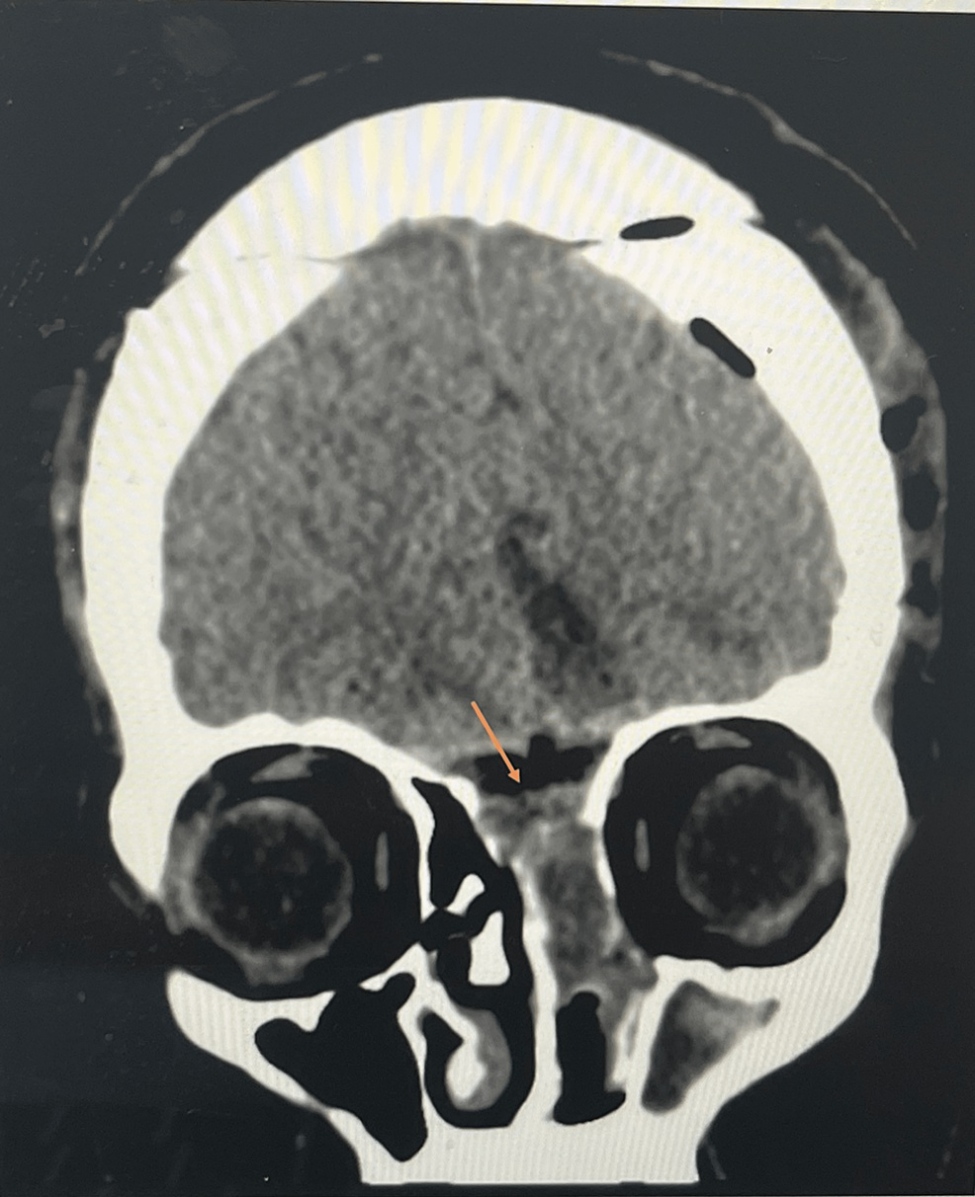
Coronal brain computed tomography without contrast.
